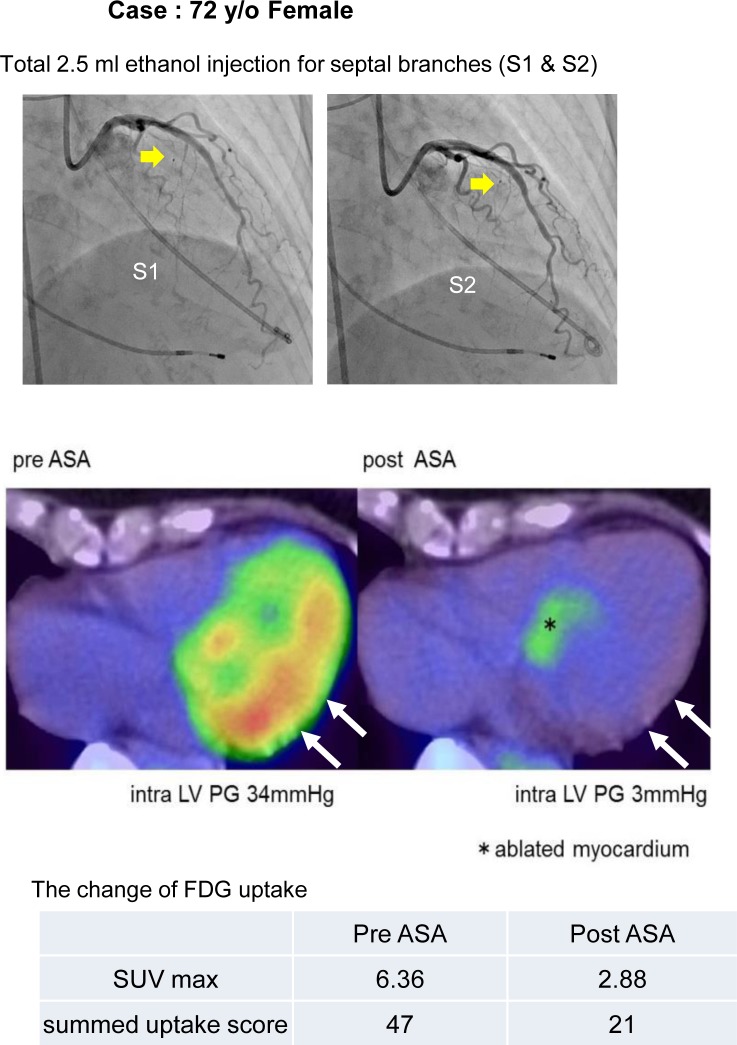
A representative case of ASA. Images of serial ^18^F-FDG-positron emission tomography (PET)/computed tomography (CT) in a representative case of an HOCM patient who received alcohol septal ablation therapy (ASA). Ablated septal branches are indicated with yellow arrows in the upper panels. In the middle panels, long axis trans-vertical LV views of ^18^F-FDG-PET /CT before and after ASA are shown. An ablated myocardium is indicated with a star symbol, and the portion with a significant reduction of ^18^F-FDG uptake in the lateral and posterior LV wall is indicated with white arrows. In the lower table, the changes of the maximum standardized uptake value (SUV max) and summed uptake score are shown.
